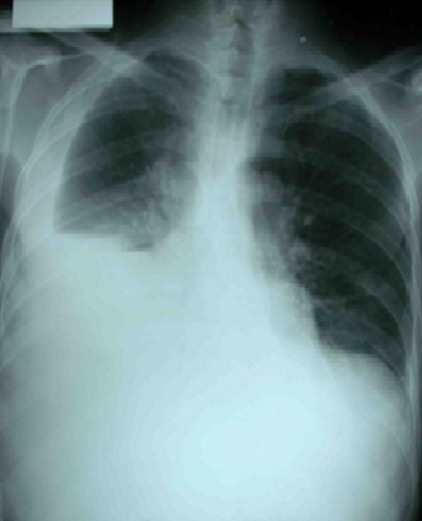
Radiographie thoracique montrant une opacité de tonalité hydrique basale droite et des images nodulaire parenchymateuse
